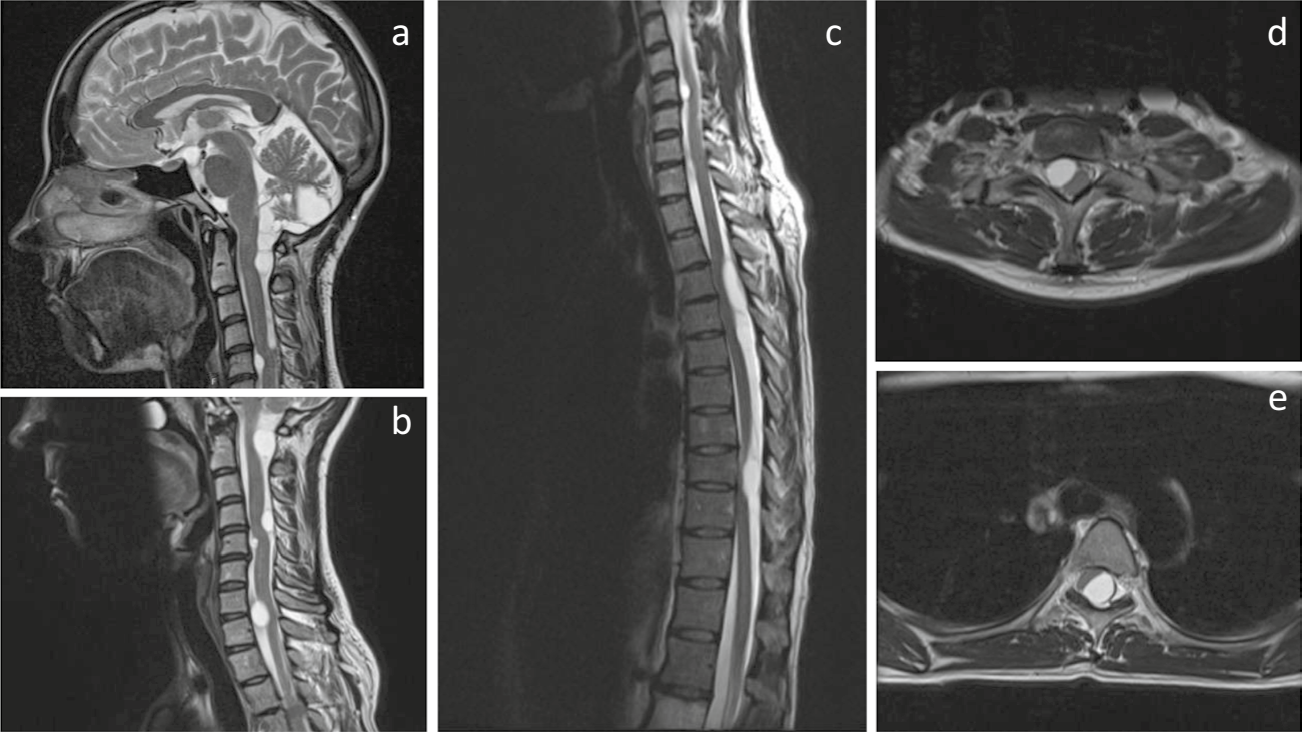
Sagittal MRI sections of the brain (a), cervical (b), and whole spine (c) as well as axial MRI at the C4 (d) and Th4 levels (e) on presentation at the neurosurgical department
